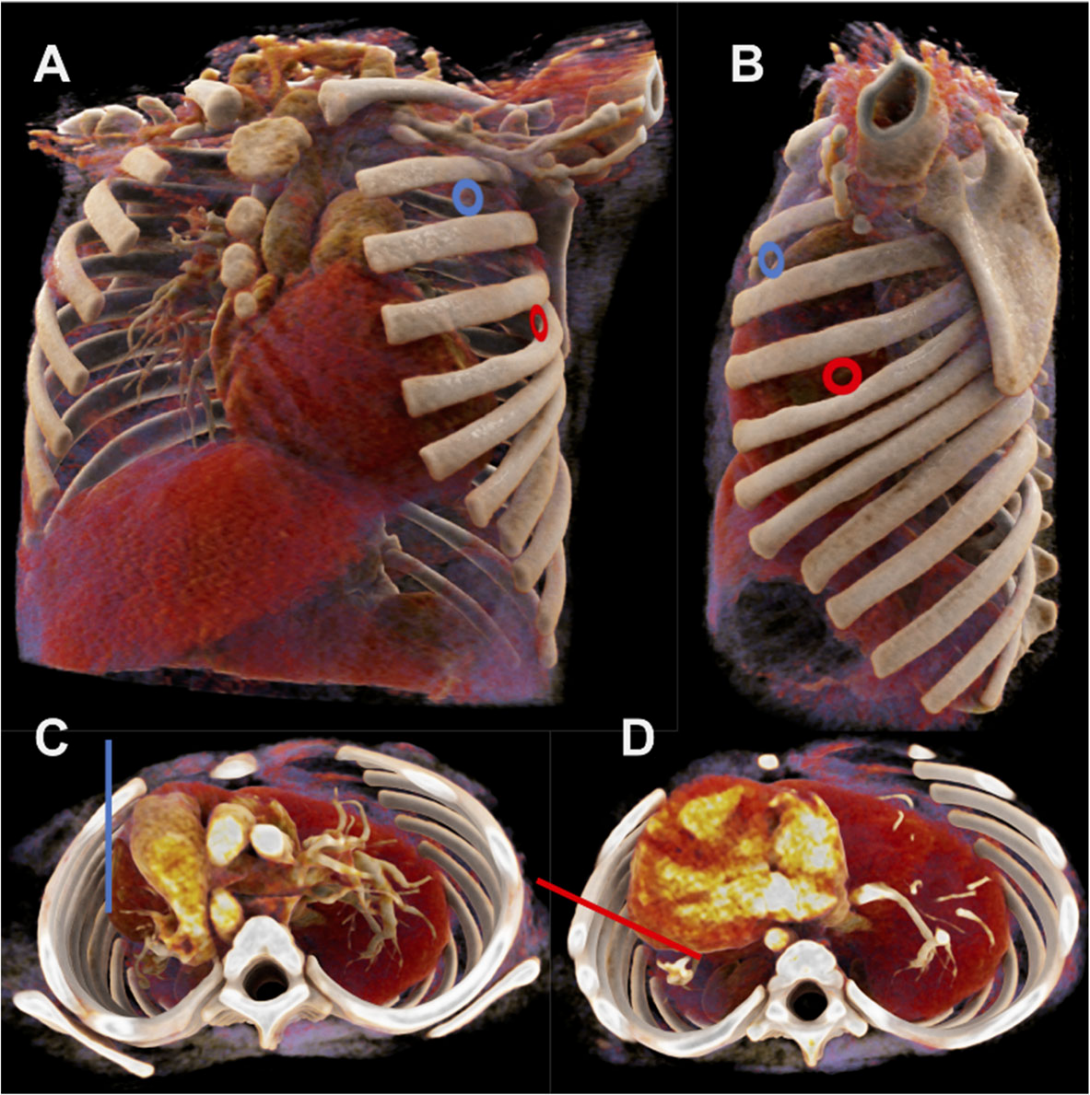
Possible insertion sites and intrathoracic structures. Reconstruction of a thoracic CT of a 21 months old girl. a and b: Insertion sites at 2nd ICS MCL and 4th ICS AAL. c: 2nd ICS midclavicular line, sagittal insertion. View from apical. d: 4th ICS anterior axillary line, insertion perpendicular to the chest wall. View from apical
